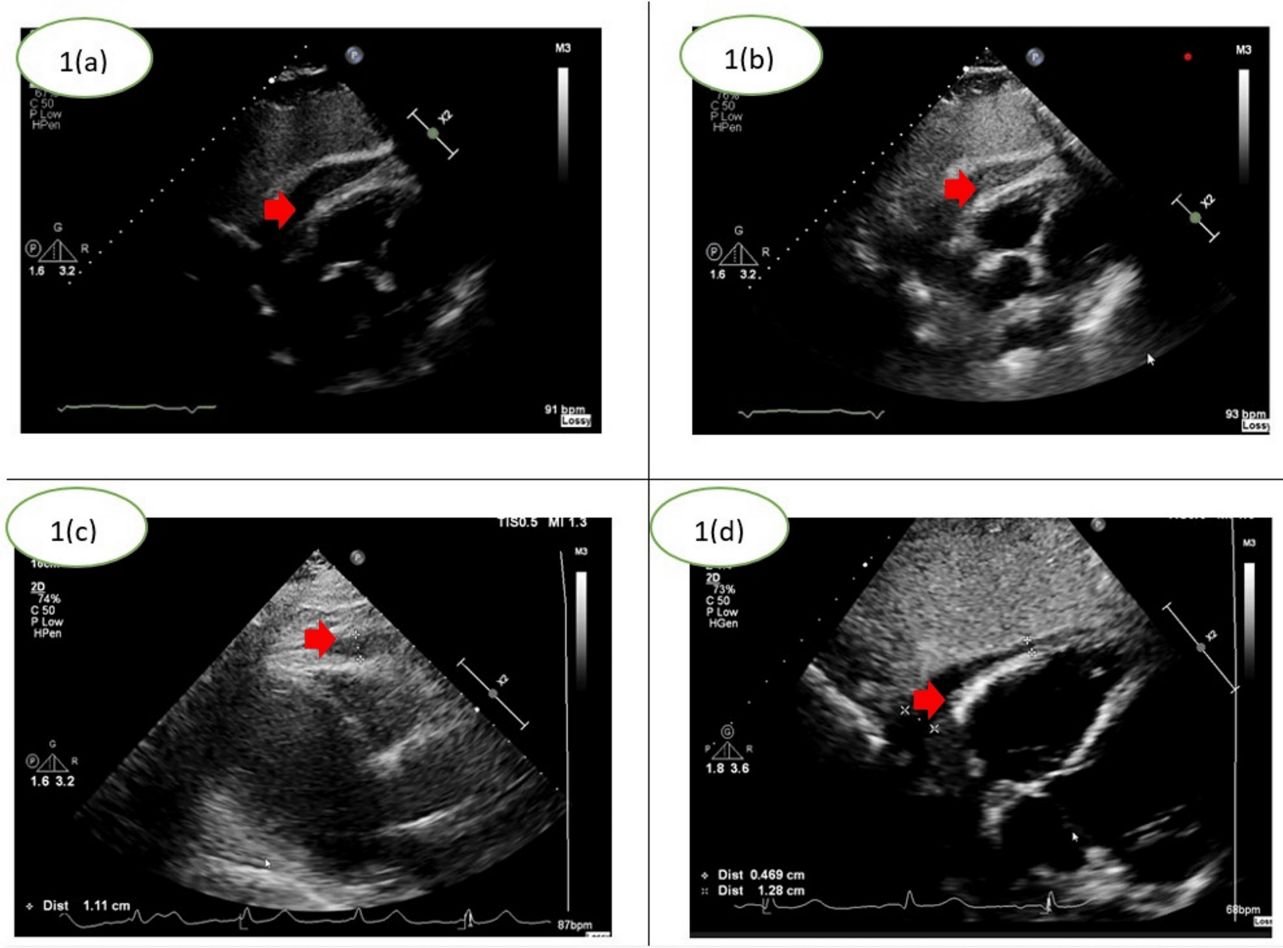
Subcostal transthoracic echocardiography (TTE) views of the pericardial effusion. 1(a)- Initial TTE showing small pericardial effusion after percutaneous coronary intervention.
